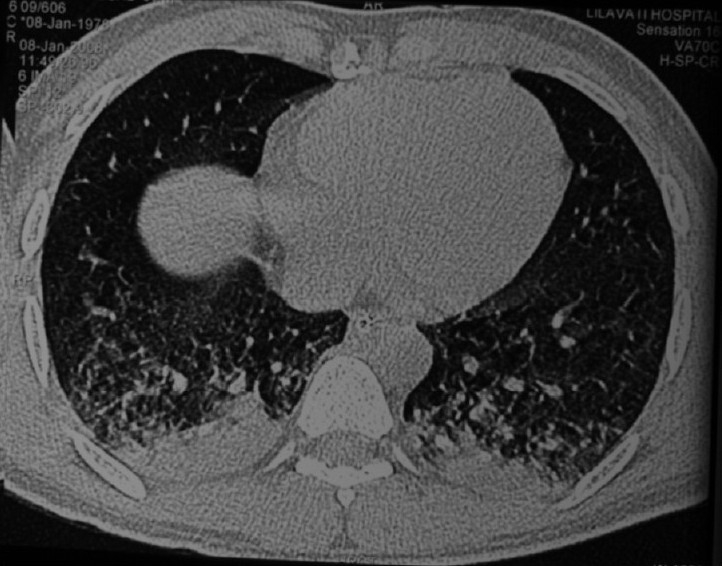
CT scan revealing B/L interstitial opacities with air trapping and with right upper lobe consolidation
